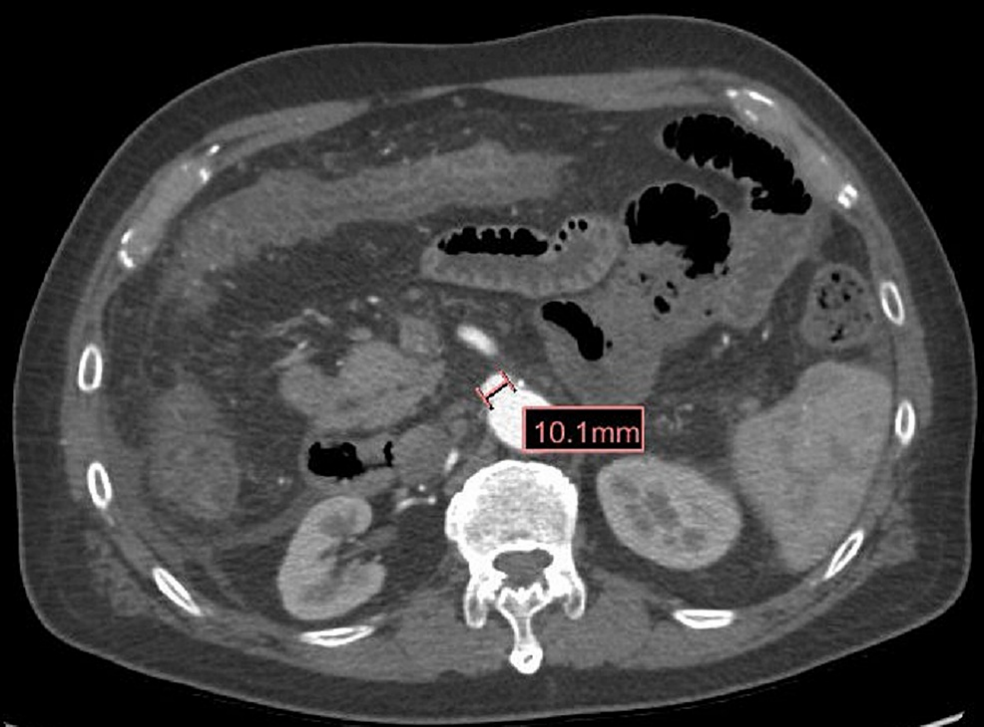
CT angiogram aorta demonstrating saccular aneurysm measuring 1 cm at origin of superior mesentric artery
